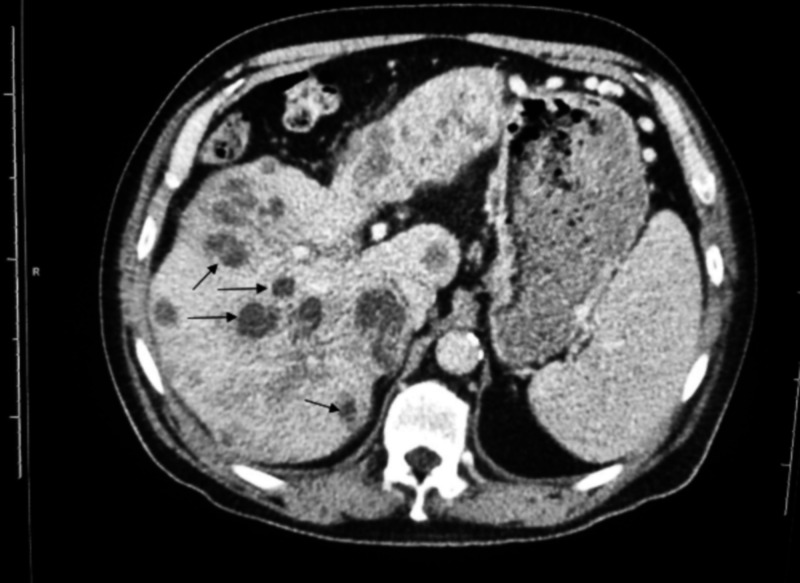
Axial view CT scan of the abdomen and pelvis with contrast shows numerous peripherally enhancing, centrally hypoattenuating masses in liver segments II, III, and IV.
